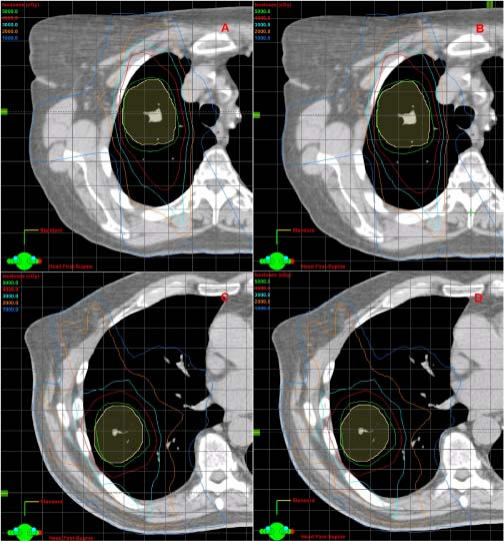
Planning CT images with isodose lines for two patients. Images A and B are from patient 1, C and D from patient 2. Images A and C show plans with flattened 6 MV beams while B and D show plans with unflattened 6 MV beams. Isodose lines represent planned doses of 50 Gy (green), 40 Gy (red), 30 Gy (cyan), 20 Gy (orange), 10 Gy (blue). The maximum dose in these plans is less then 60 Gy. The PTV is shown in yellow.
